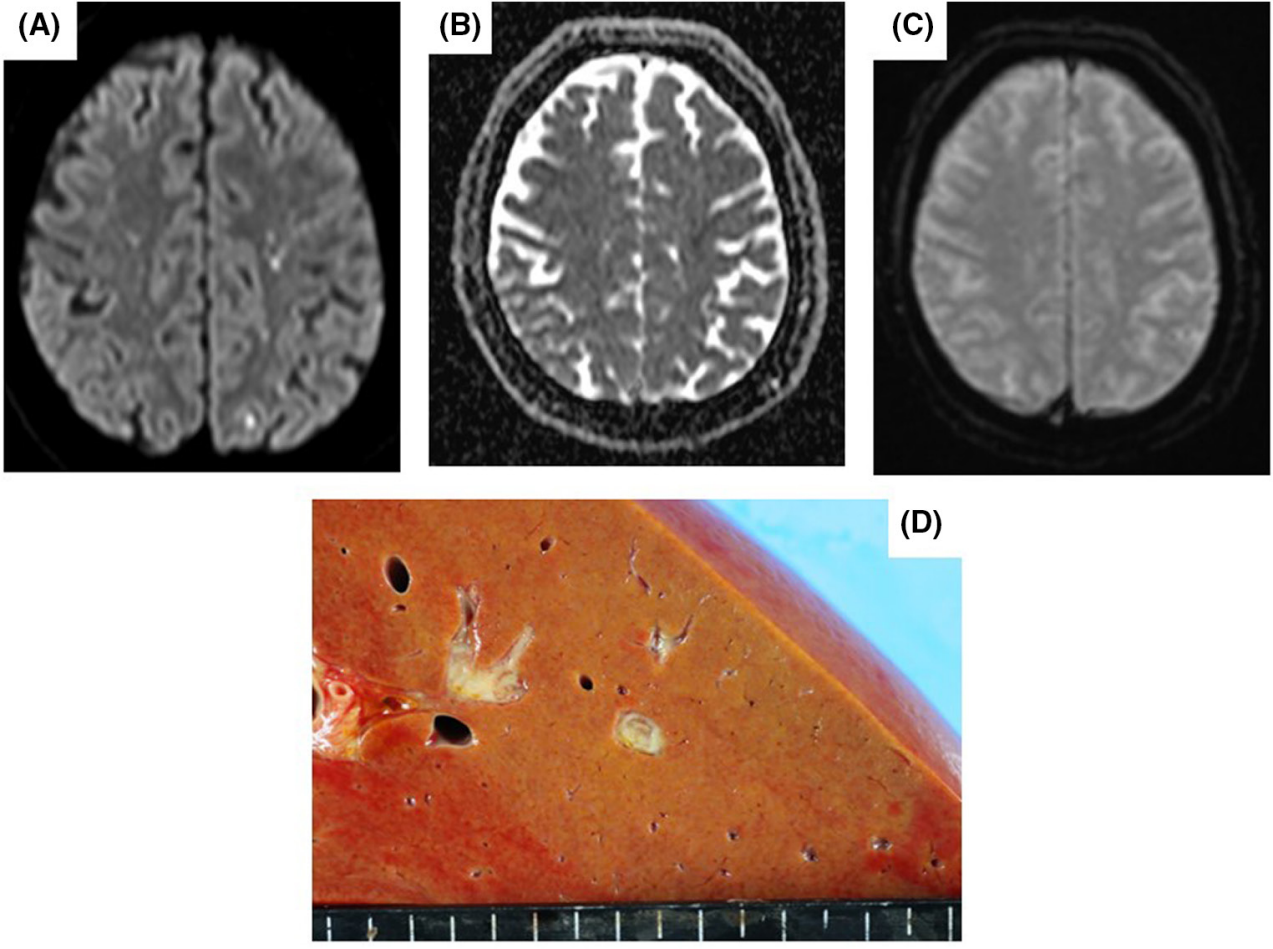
(A–C) Magnetic resonance imaging scans in Case 1 showing multiple foci of restricted diffusion in the cortimomedullary junction. (A) The diffusion‐weighted image. (B) The apparent diffusion coefficient image. (C) The T1‐weighted image. (D) Autopsy investigation findings in Case 1 showing a small abscess in the liver.
